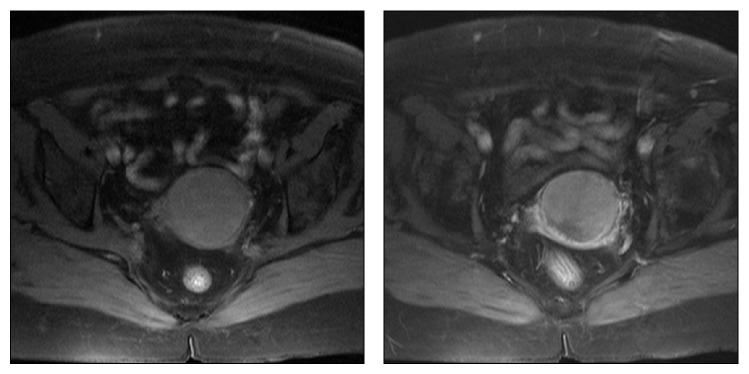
Pelvic MRI demonstrates a mass of malignant melanoma in size of 5.5 × 7.5 cm in uterine cervix area.
